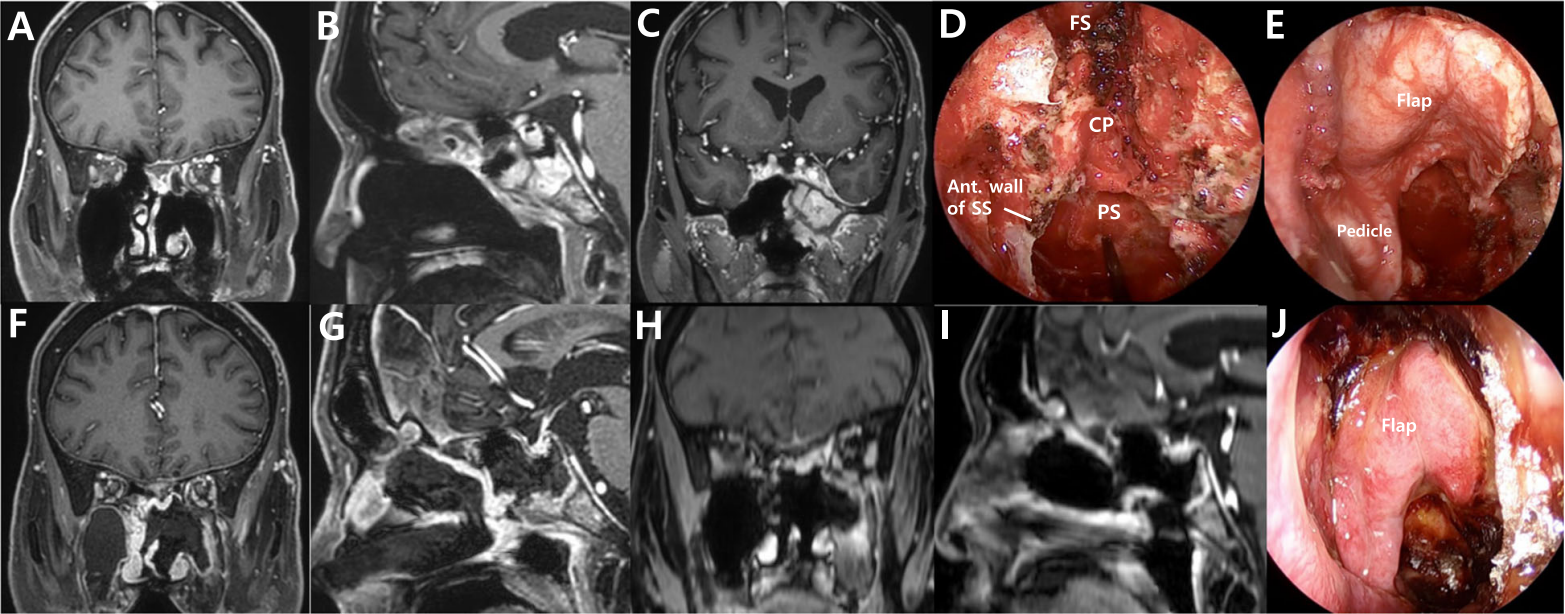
Images from Case 1. A recurrent olfactory neuroblastoma. a Coronal enhanced magnetic resonance imaging (MRI) reveals a recurrent tumor on the frontal base, which was operated upon by an endoscopic endonasal approach from the left nostril. b Enhanced sagittal MRI shows a mass with irregular margins in the left ethmoid sinus extending to the frontal base bone, dura, and sphenoid sinus. c The tumor can be seen extending into the left side of the sphenoid sinus and nasal cavity. d The right lateral side of the sphenoid sinus wall is left intact to support the pedicle of the PNSF. e The PNSF covers the ASB defect through the bony support of the remnant lateral side of the sphenoid sinus wall. f, g Postoperative coronal and sagittal MRI reveals a well enhanced PNSF and coverage of the ASB defect. h, i One year after the operation, the follow-up coronal and sagittal MRI showed well-healing state of the nasal mucosa and no recurrence of the tumor. j An endoscopic view shows a well-harvested PNSF at 2 weeks after the operation. FS: frontal sinus, CP: cribriform plate, SS: sphenoid sinus, PS: planum sphenoidale, PNSF: pedicled nasoseptal flap, ASB: anterior skull base
